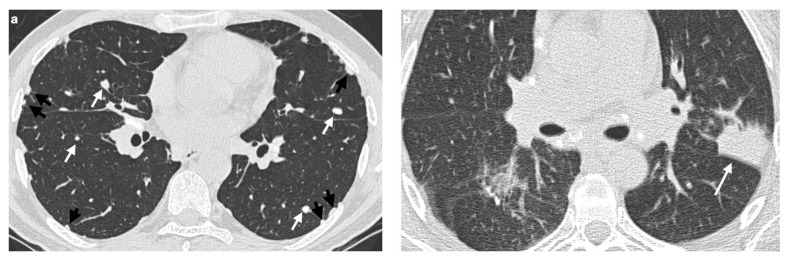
(a) Chest CT scan demonstrates the presence of parenchymal nodules (white arrows) and multiple nodules in the subpleural areas of both lungs (black arrows). (b) in a patient with known sarcoidosis, it is possible to recognize an area of consolidation in upper left lobe (white arrow); bilateral lymphnodal calcification in the mediastinum can also be noted.
